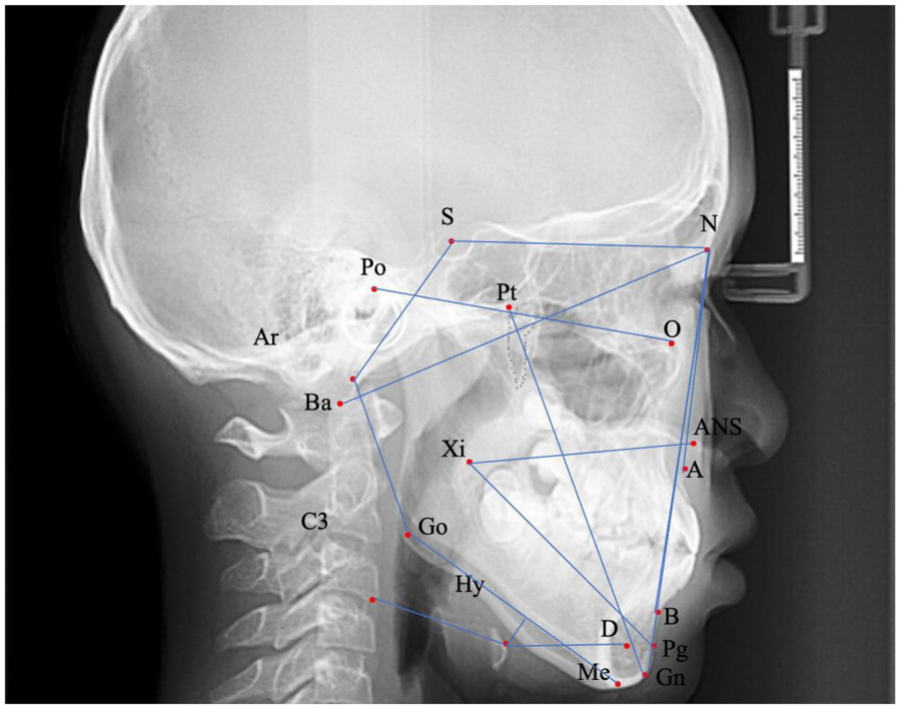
Cephalometric landmarks and reference points used in this study. Lateral cephalometric radiograph demonstrating all anatomical landmarks employed for measurement and analysis. Key landmarks are labeled as follows: N, nasion; S, sella; ANS, anterior nasal spine; A, subspinale (Point A); B, supramentale (Point B); Ar, articulare; Ba, basion; Pt, pterygomaxillary fissure; Po, porion; O, orbitale; Xi, xi point (mid-ramus); Go, gonion; Hy, hyoid bone; D, point D (mandibular landmark); Me, menton; Pg, pogonion; Gn, gnathion; C3, third cervical vertebra. Blue lines connect the key points according to measurement protocols. These landmarks correspond to the definitions and measurement items described in Table 1.
